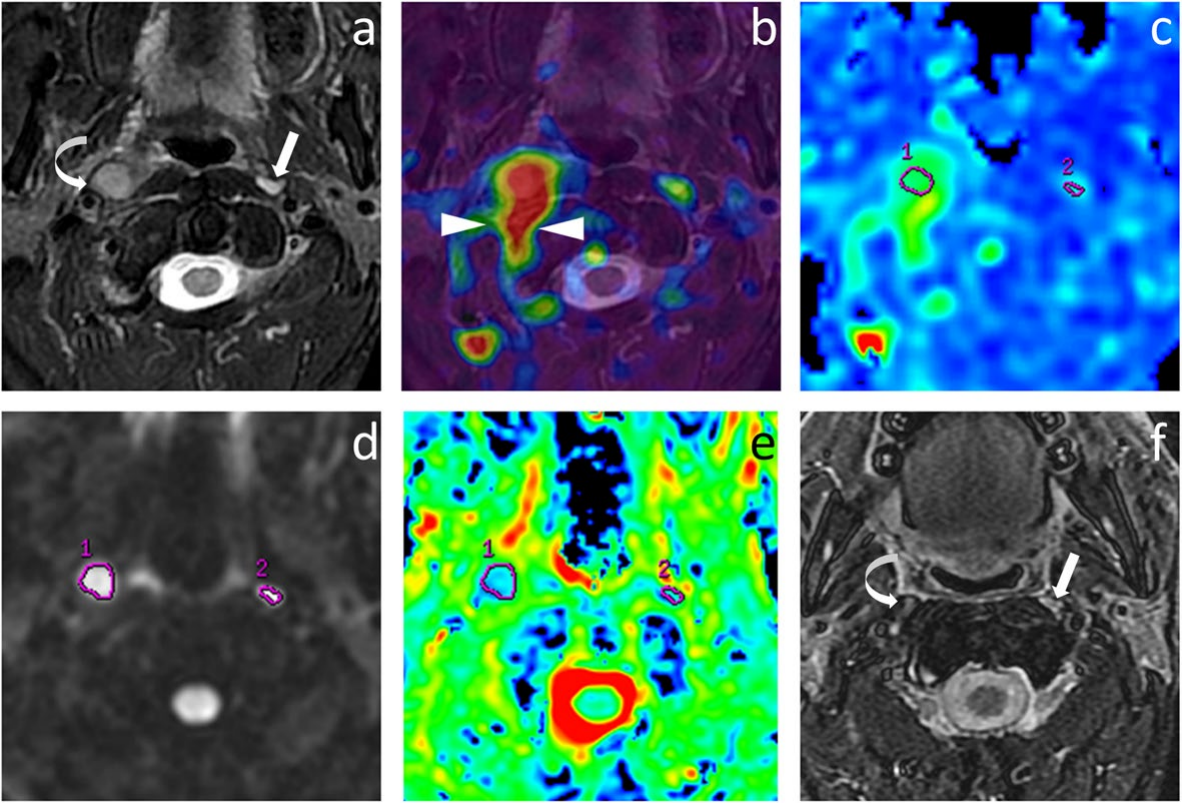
M50, NPC with right metastatic RLN, and left non-metastatic RLN. Axial T2WI before treatment (a) showed bilateral RLNs with slightly high heterogeneous signal intensity and size as long axis × short axis of 19 × 11 mm (right, curved arrow) and 7 × 5 mm (left, straight arrow), respectively. Both lymph nodes demonstrated higher perfusion than the surrounding tissue on the fused image by T2WI and ASL (b), and the map of BF (c), especially the right one with remarkable pseudo-color contrast. On the fused image (b), the high perfusion area behind the right lymph node (arrowhead) was the internal carotid. ROI of the lymph nodes were delineated on the map of BF (c) and multi-b-value DWI with b value = 800 s/mm^2^
